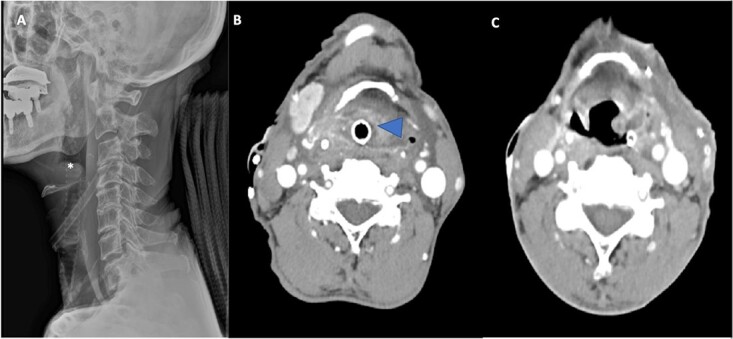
(A) Lateral radiograph of the neck shows a swollen epiglottis (^*^). (B) Computed tomography axial cut shows a collection in the paraglottic space (arrowhead) and parapharyngeal space extenuation with effaced pharyngeal and supraglottic lumina due to epiglottic enlargement. (C) Computed tomography axial cut at Day 7 post-operatively reveals a marked reduction in the size of the collection.
